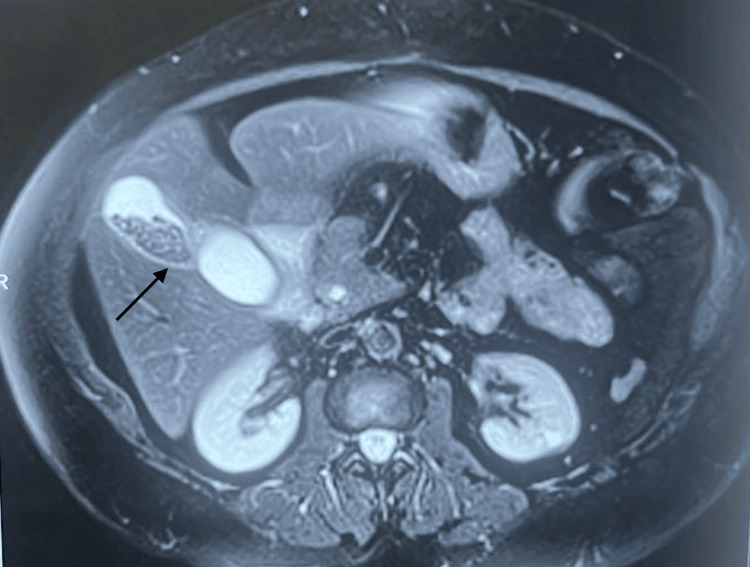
MRI image of the duplicated gallbladder with stones in one of the lobes
